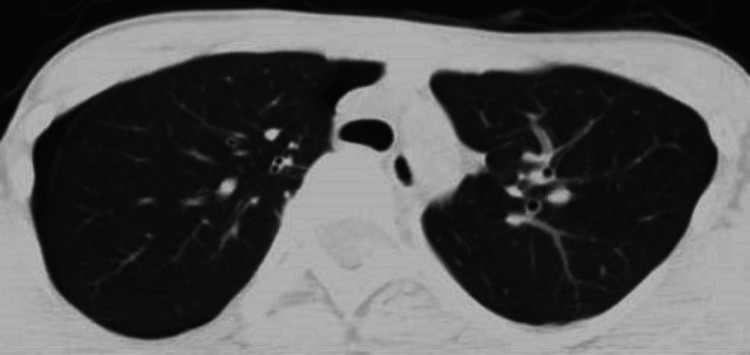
Preoperative computed tomography. Pectus excavatum and curved spine due to scoliosis seemed to have compressed the airway to some extent.
